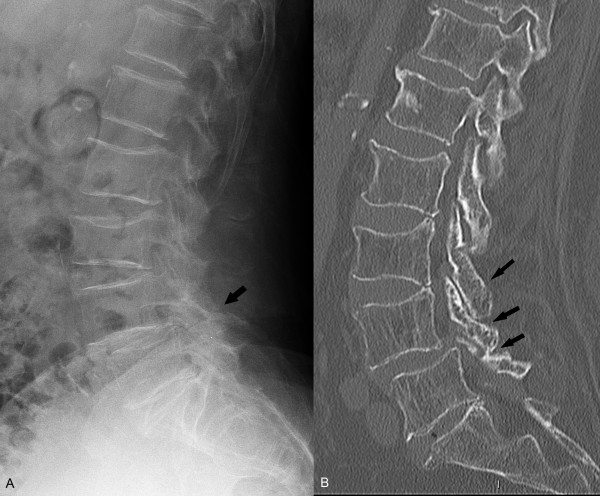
Degenerative Lumbar Spondylolisthesis (DLS). A lateral radiograph (A) and sagittal reconstructed CT image (B) of this 78 year old woman shows a 10 % anterolisthesis of L4 associated with severe facet joint osteoarthrosis (arrows). In this patient, severe osteoporosis has led to a fragility fracture of the L3 vertebral body. (Images courtesy of Lindsay J. Rowe, Newcastle, Australia).
